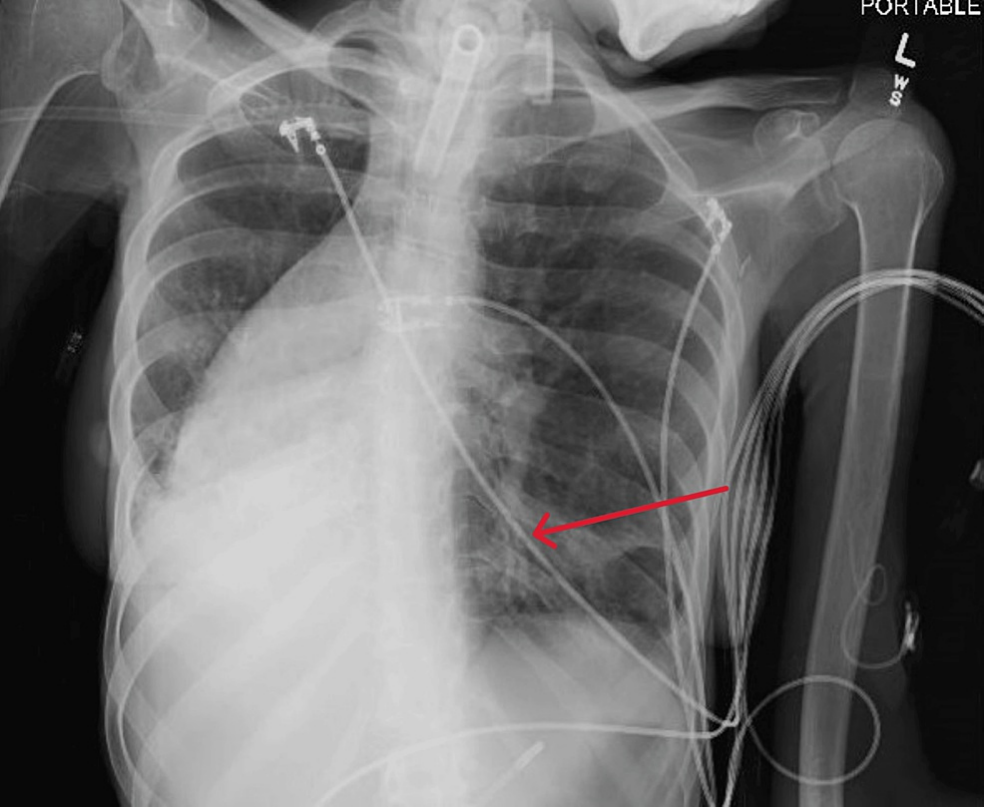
Chest X-ray showcasing a basilar opacity in the left lower lobe (red arrow) and right-sided mediastinal shift.
